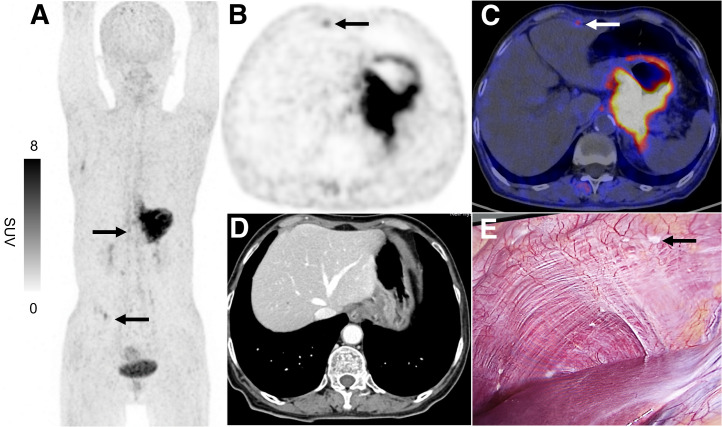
^68^Ga-FAPI-04 PET images of 71-y-old woman with poorly differentiated gastric adenocarcinoma and peritoneal carcinomatosis. (A) Maximum-intensity-projection image demonstrated multiple foci in upper and right lower abdominal quadrants (arrows; SUV_max_, 4.17). Axial PET (B) and PET/CT fusion (C) images localized upper abdominal focus to left subdiaphragmatic space (arrows), with no corresponding abnormality on contrast-enhanced CT (D). (E) Subsequent preoperative staging laparoscopy confirmed tiny implants on diaphragmatic surface (arrow).
